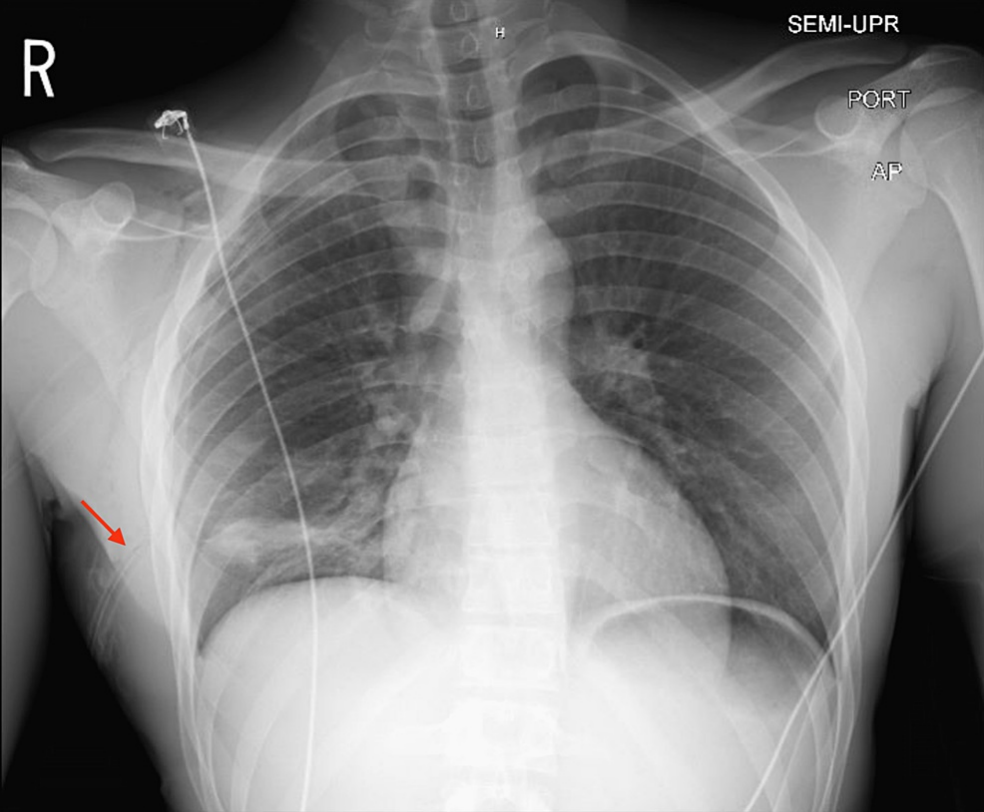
Chest x-ray after right chest tube placement
